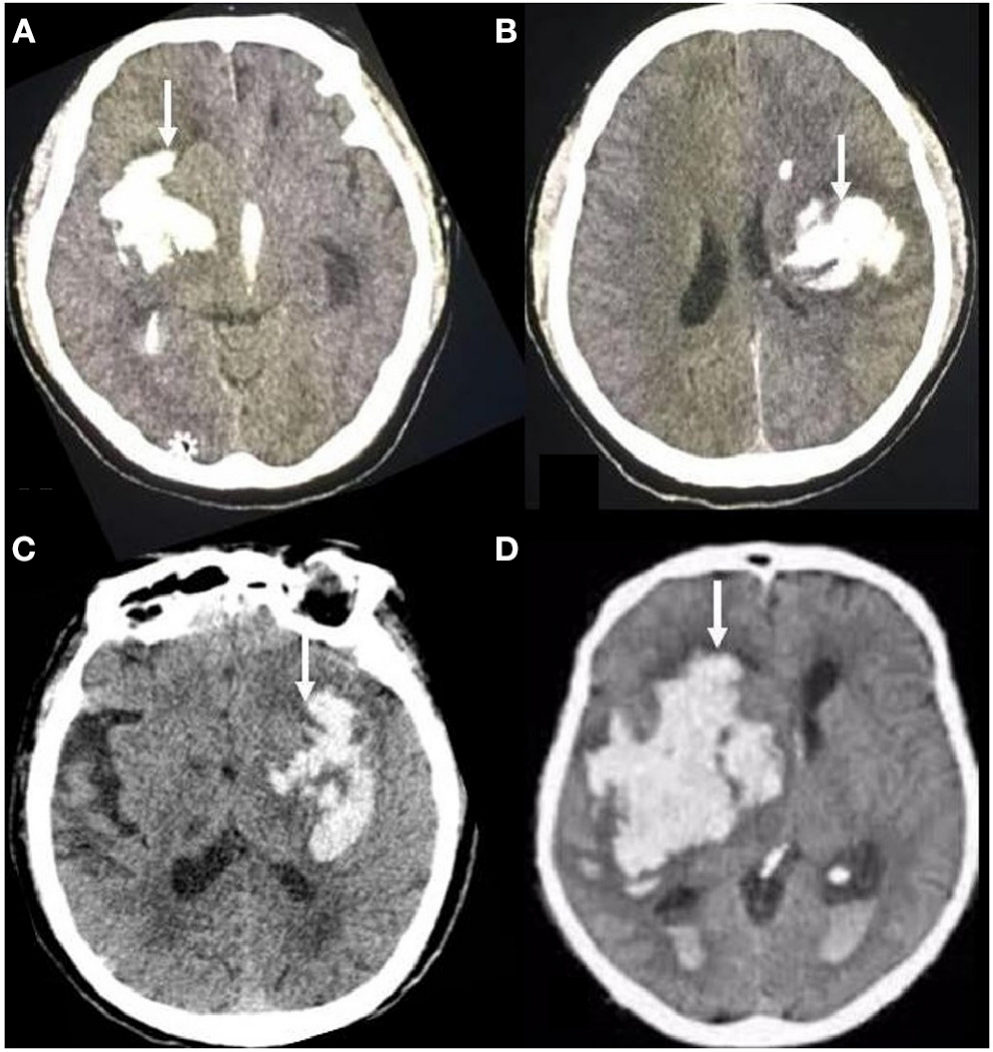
Brain transverse CT section showing examples of hematoma shape. On the transverse CT scans of the brain, the irregular shape of hematoma showed different features, corresponding to grades III (A, B) or IV-V (C, D) of the Barras scale.
